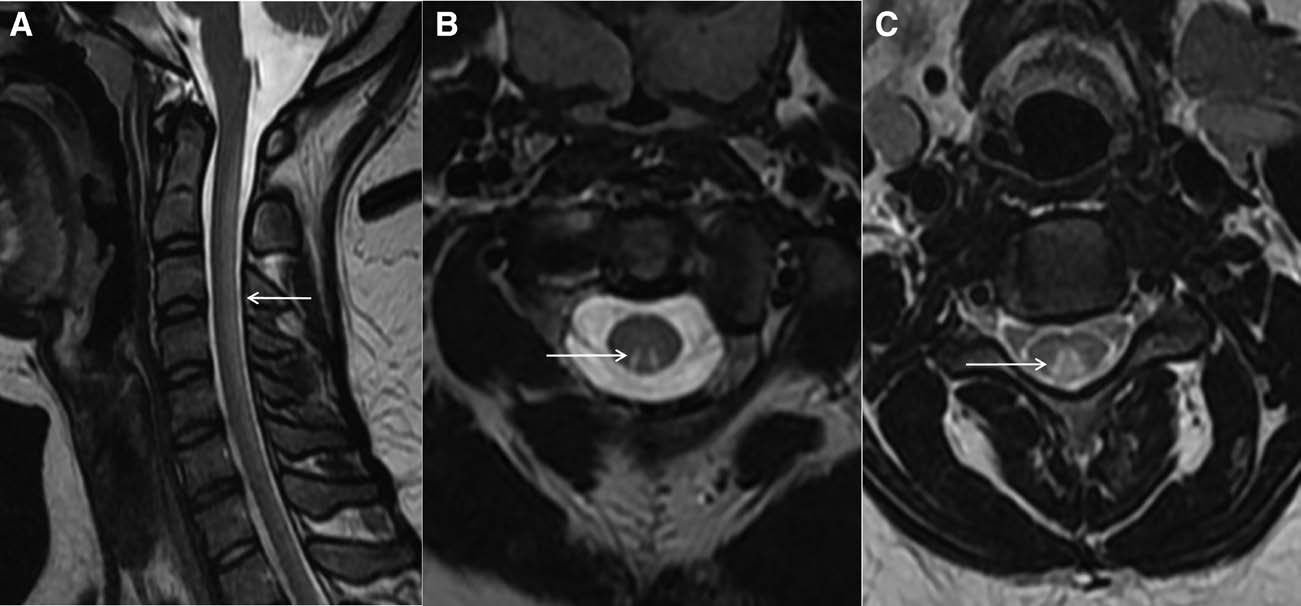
An 18-yr-old woman with subacute combined degeneration of the spinal cord due to nitrous oxide abuse in the first hospitalization. The sagittal T2-weighted images show a high signal along the posterior column of the cervical spinal cord (A, white arrows). Axial T2-weighted images demonstrate hyperintense signal in the dorsal columns of the cervical cord with the “inverted V” sign (B, white arrow) and the “triangle” sign (C, white arrow).
